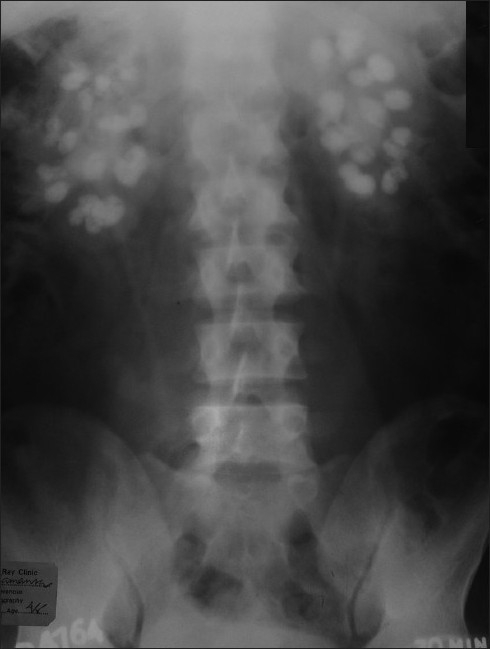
Photograph shows straight X-ray of abdomen with bilateral extensive nephrocalcinosis.
